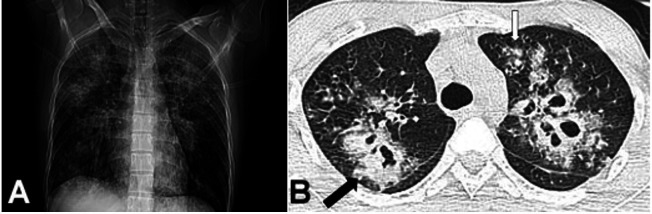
A – Chest x-ray showing bilateral heterogeneous opacities in the pulmonary parenchyma; B – Thoracic computed tomography showing bilateral upper lobe nodules of varying size and multiple cavitations. One of the them shows intracavity content (black arrow). Ground glass opacities surrounding a few nodules in the left upper lobe (halo sign; white arrow).
